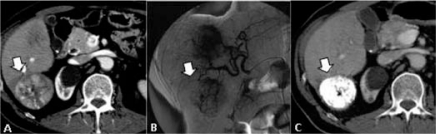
(a) pre-TACE CT shows a huge hypervascular nodule of HCC located into the segment VI; (b) hepatic angiogram detects many arteries feeding the lesion to be treated; (c) post-TACE CT shows the intense Lipiodol uptake into the treated lesion, which is a little bit smaller after one month.
